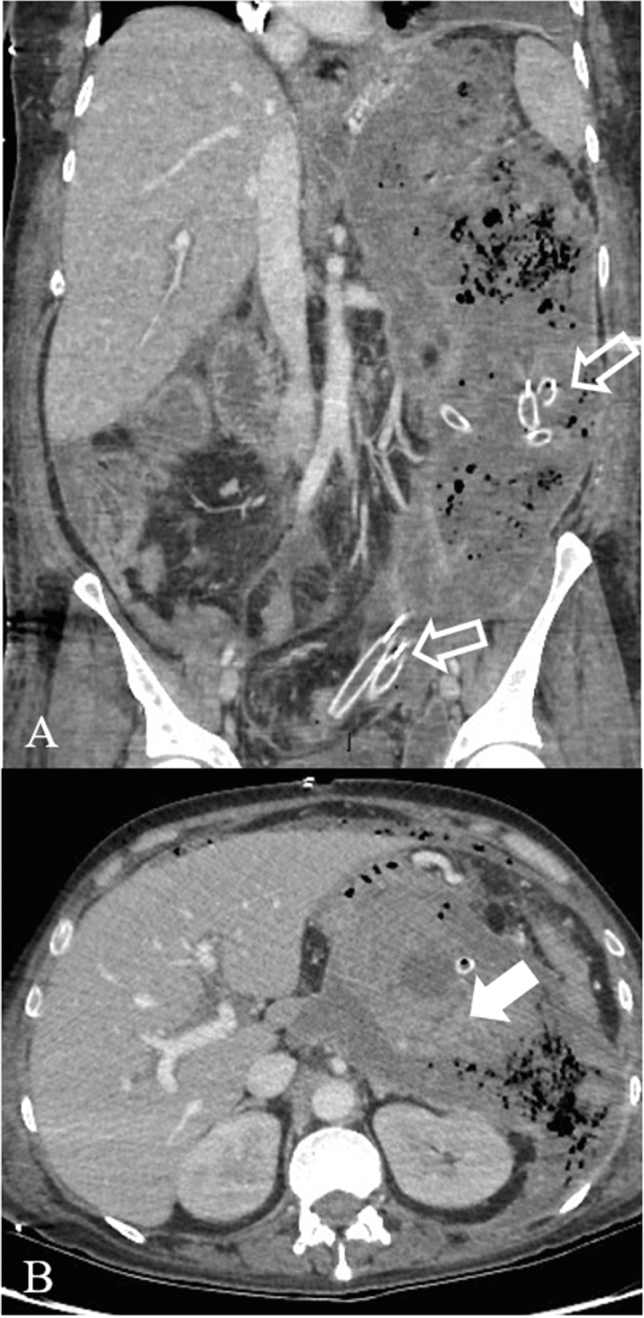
Necrotizing Pancreatitis. The same case as the previous in Fig. 3, after 1 month of the onset of the symptoms, and 1 week after surgical necrosectomy, the contrast-enhanced CT scans in the portal phase show a peri-pancreatic collection with bubbles air and drainage tubes in the collection (empty arrows). Coronal MPR image (a) shows the extention of the collection. Axial scan (b) show diffuse alterations of the CE of the parenchyma, due to necrosis (arrow)
